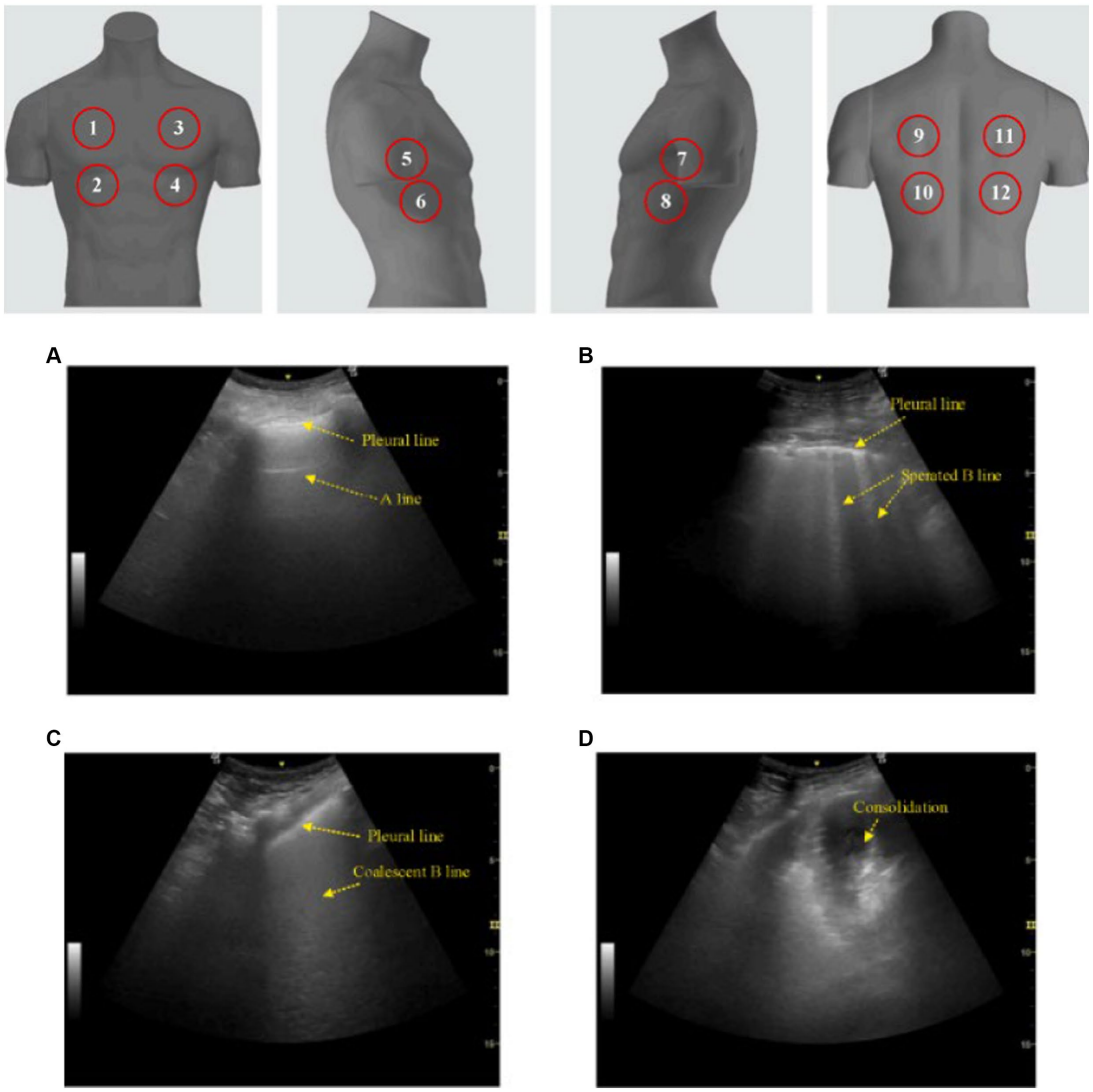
Lung ultrasound score (LUS). The classic LUS requires scanning 12 zones (upper image; 6 on each hemithorax) while scoring each zone from 0 to 3 based on pattern. (A) A score of 0 is a normal pattern. (B) A score of 1 requires at minimum 3 isolated or coalescent B-lines covering <50% of the screen without clear subpleural alterations. (C) A score of 2 is given when B-lines encompass >50% of the screen without clear subpleural alterations. (D) And finally, a score of 3 is made when consolidation is observed. Total scores range from 0 to 36. This image is reproduced with permission from the Elsevier Novel Coronavirus Center. No changes were made to this image (38).
